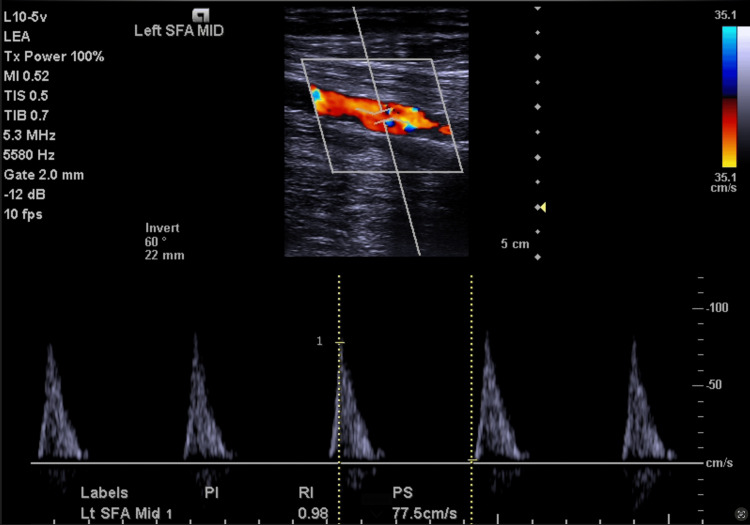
Arterial ultrasound revealed monophasic to biphasic waveforms on the left side with moderate infrapopliteal disease and decreased velocity
